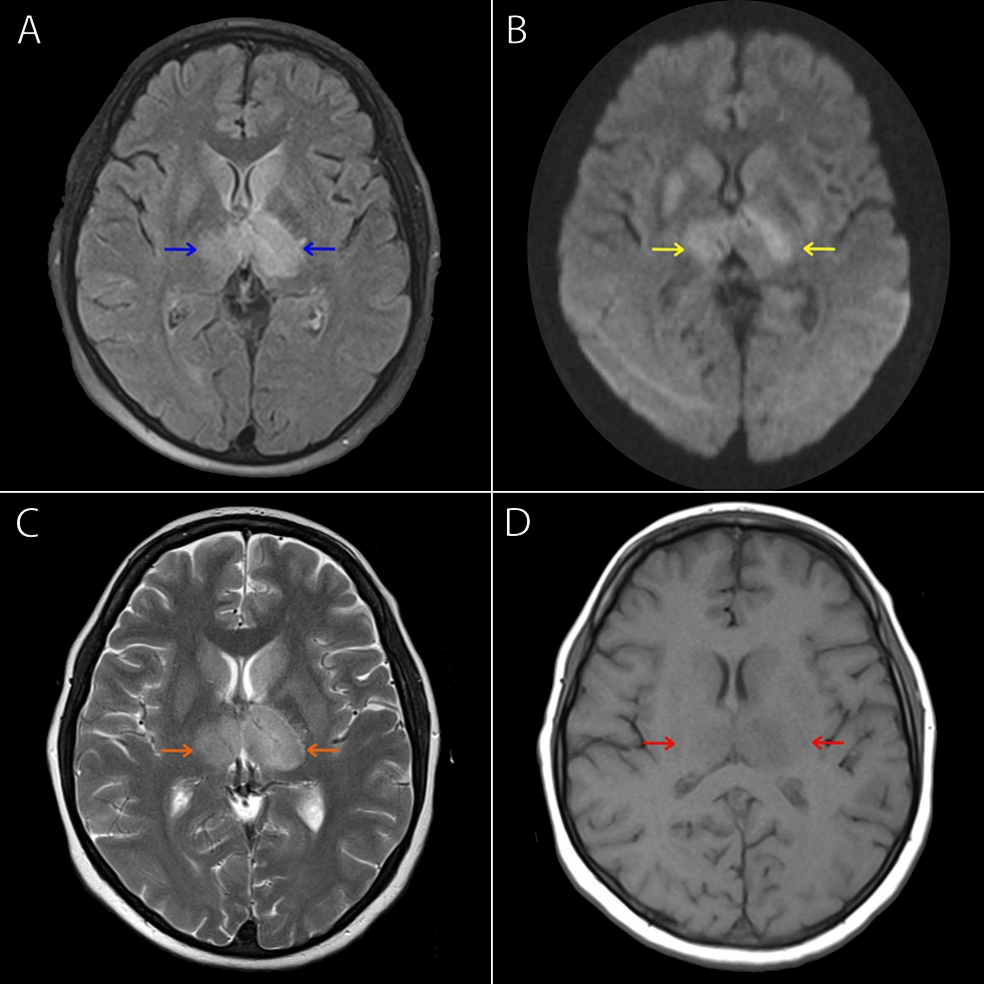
Multi-sequence MRI of the brain showing thalamus depicted in (A) T2 dark fluid sequence (blue arrows), (B) FLAIR sequence (yellow arrows), (C) T2 sequence (orange arrows), and (D) T1 sequence (red arrows). FLAIR, fluid-attenuated inversion recovery.
